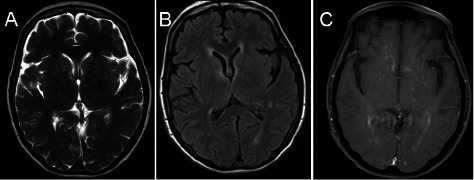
Figure 1. Axial Brain MRI sequences prior to treatment. A: T2 weighted image, B: FLAIR showing abnormal signal prolongation in periventricular areas, C: post contrast T1 image showing mild diffuse enhancement.
